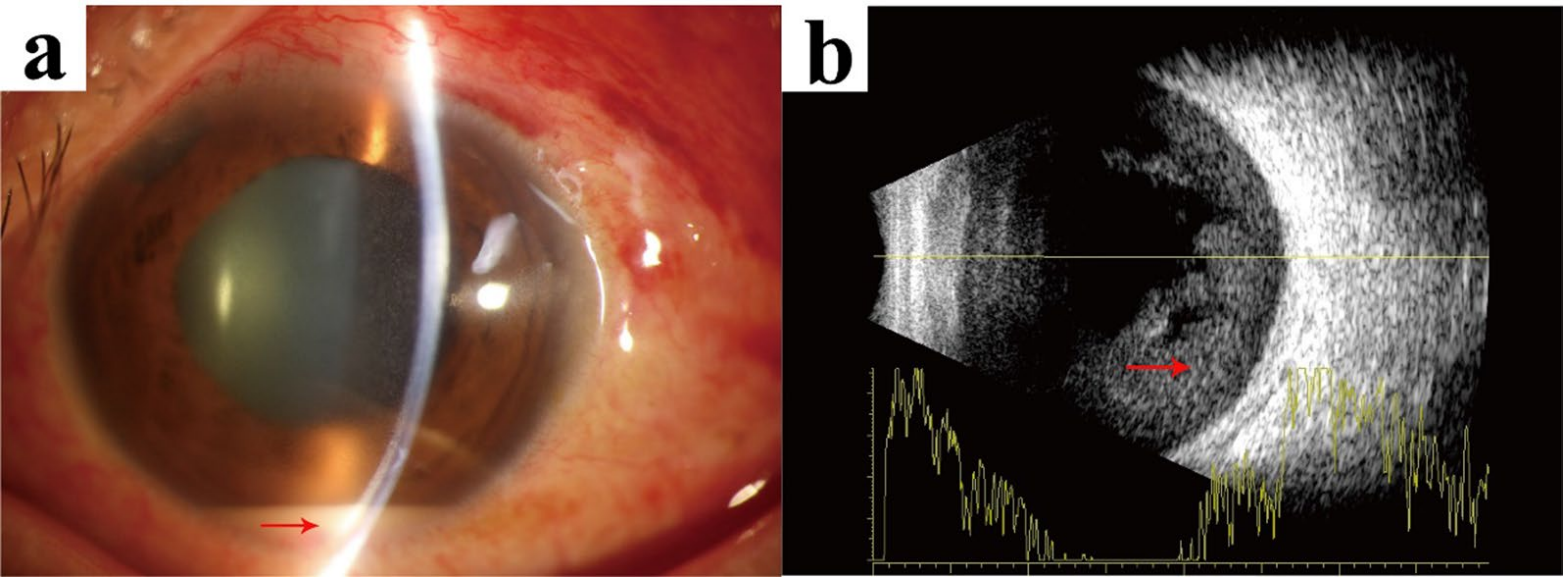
Images were obtained following the patient’s hospitalization. a: Slit lamp examination revealed a 1.5-mm hypopyon (red arrow showing hypopyon). b: A brightness scan ultrasound confirmed dense vitreous opacities (red arrow showing vitreous opacity)
